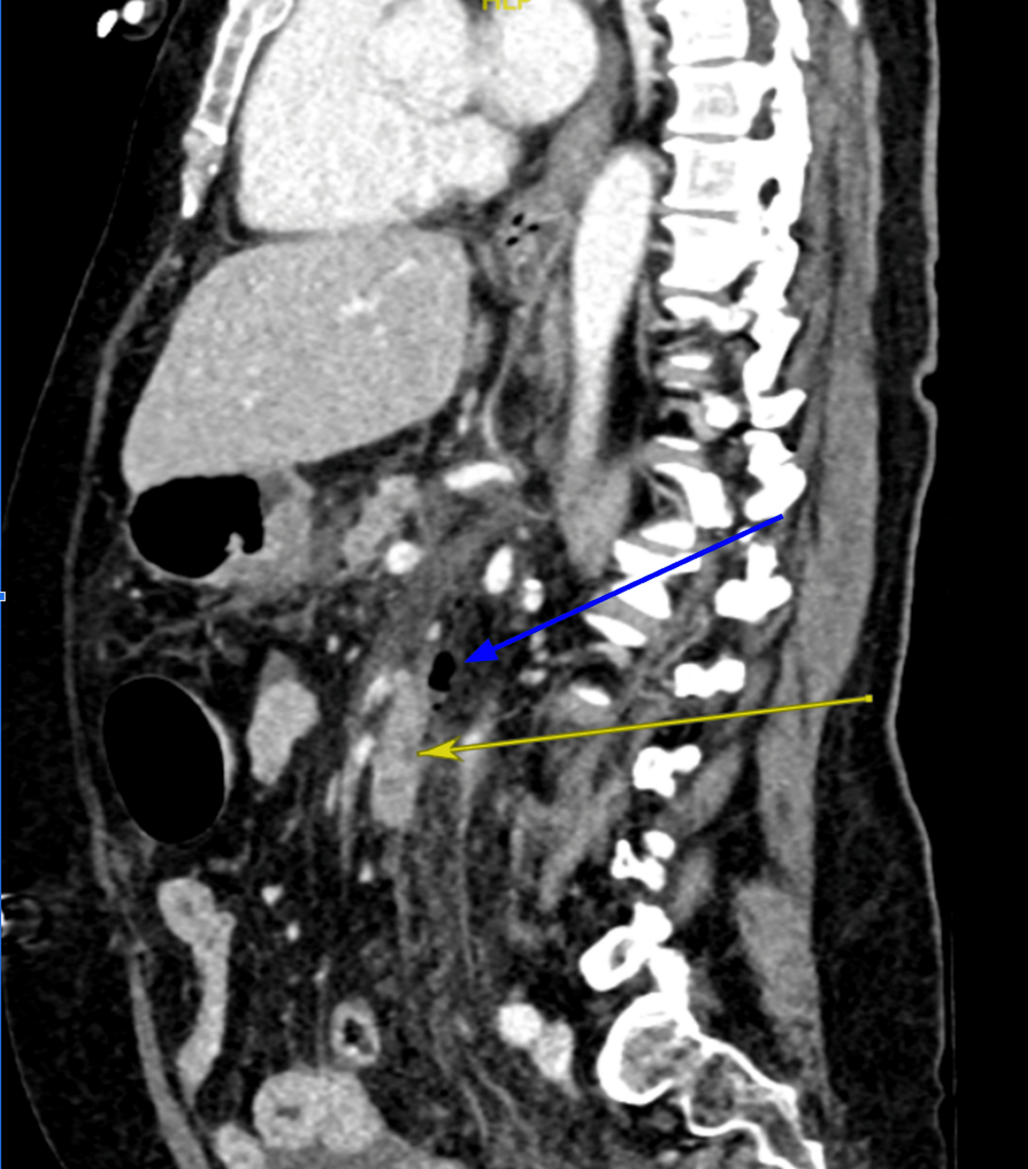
Computed tomography (CT) abdomen in sagittal view, demonstrating free intraperitoneal fluid and retroperitoneal gas adjacent to the duodenum (blue arrow) with retroperitoneal fat stranding (yellow arrow).
